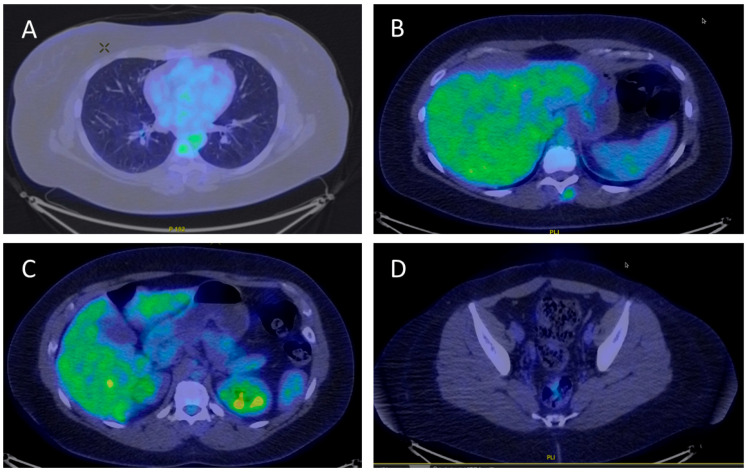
PET-CT images from 09/2024. No bright spots detected at the former metastatic sites. (A) Total regression of the pulmonary nodes. (B,C) No hepatic or splenic metastasis detected. (D) No pelvic metastasis detected. GE Signa^TM^ Explorer 1.5T (GE Health Care, 500 West Monroe Street, Chicago, IL 60661, USA) was used.
